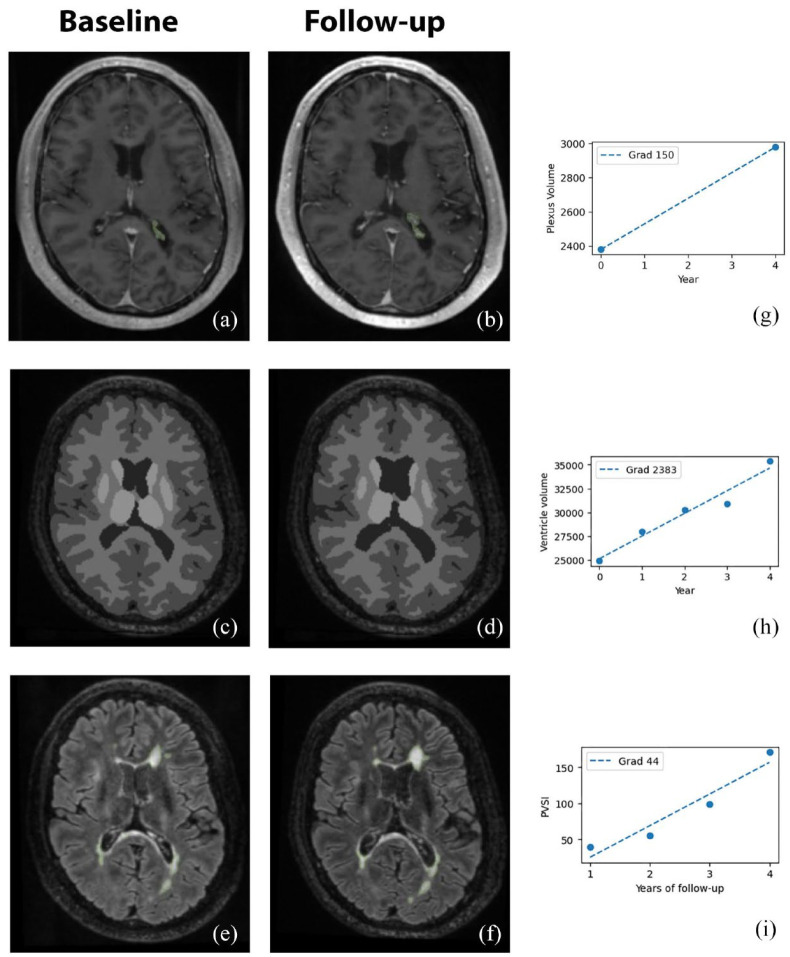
Example of choroid plexus delineation (only right side shown for clarity) using manual segmentation (a, b) (GAD 3D T1), brain tissue segmentation using AssemblyNet (c, d) (3D T1) and lesional segmentation using iQ-MS^TM^ (e, f) (FLAIR). All images presented at baseline and last follow-up. Right column shows values for plexus volume measured at baseline and last follow-up visit (g), ventricle volume values (h) and chronic lesion volume values measured annually (i). The line of best fit represents the annual gradient of change for each of those measures.
